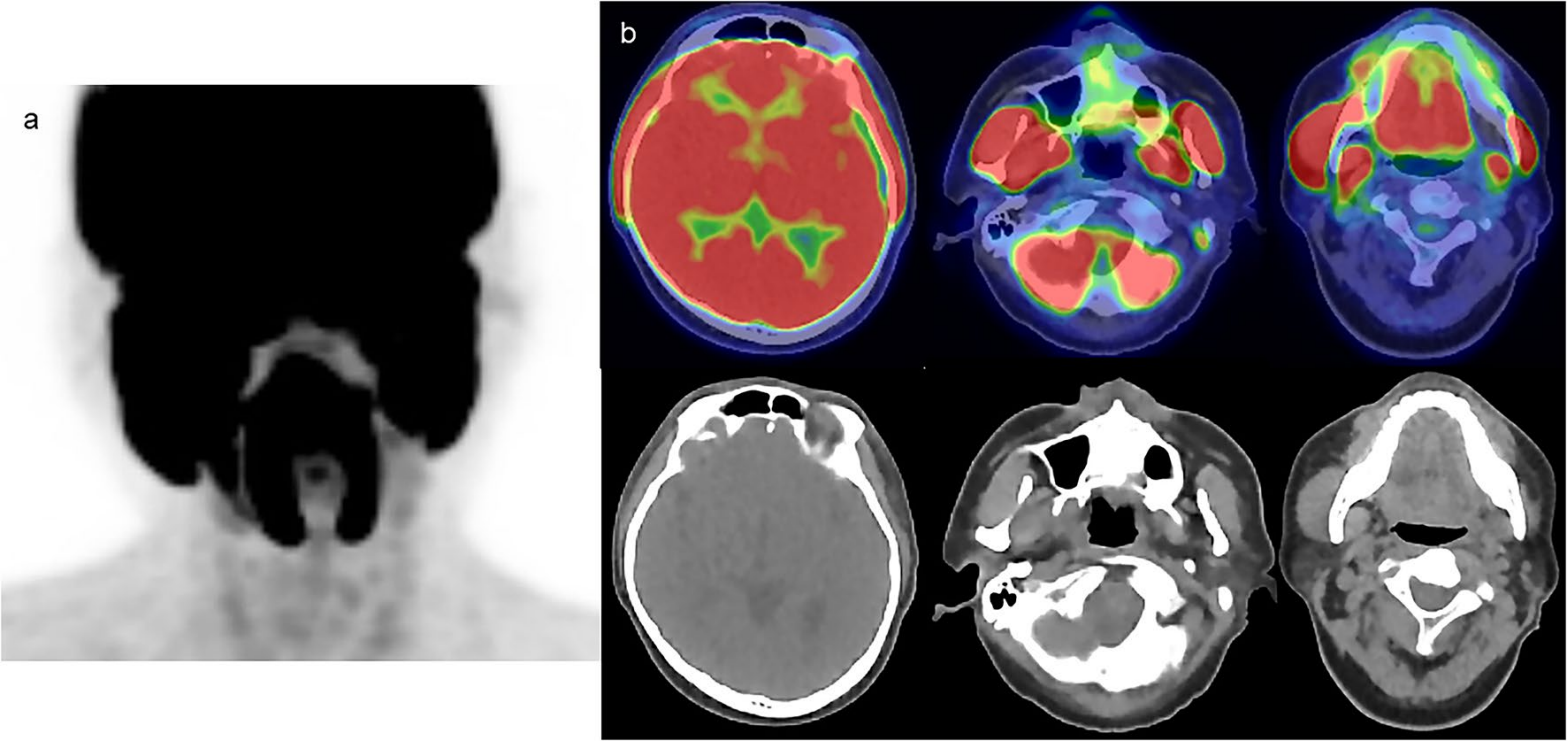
a FDG MIP image in a patient with Sjögren’s syndrome. b Upper: fusion images, lower: CT images. The high FDG uptake seen in the facial and masseter muscles is due to chewing gum
